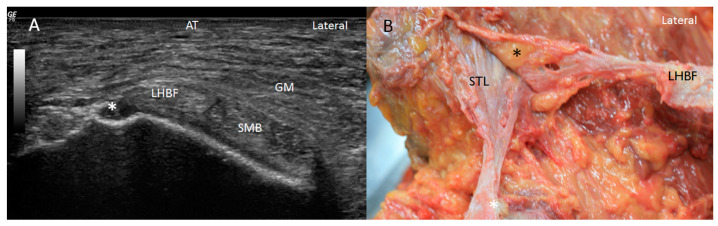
(A) Ultrasound view of the sacrotuberous ligament insertion at the vertex (white *) of the ischial tuberosity (IT) in the short axis tendon of the semimembranosus muscle (SMB) on the right side (gluteus maximus muscle). AT: Adipose tissue. (B) Anatomical dissection of the sacrotuberous ligament on the right side showing the possibility to separate different layers of the STL. The most superficial layer of the STL (black *) follows with the LHBF, and it is separated from the deep layer with adipose tissue (black *) and other layers of the STL inserted at the vertex of the IT (white *).
